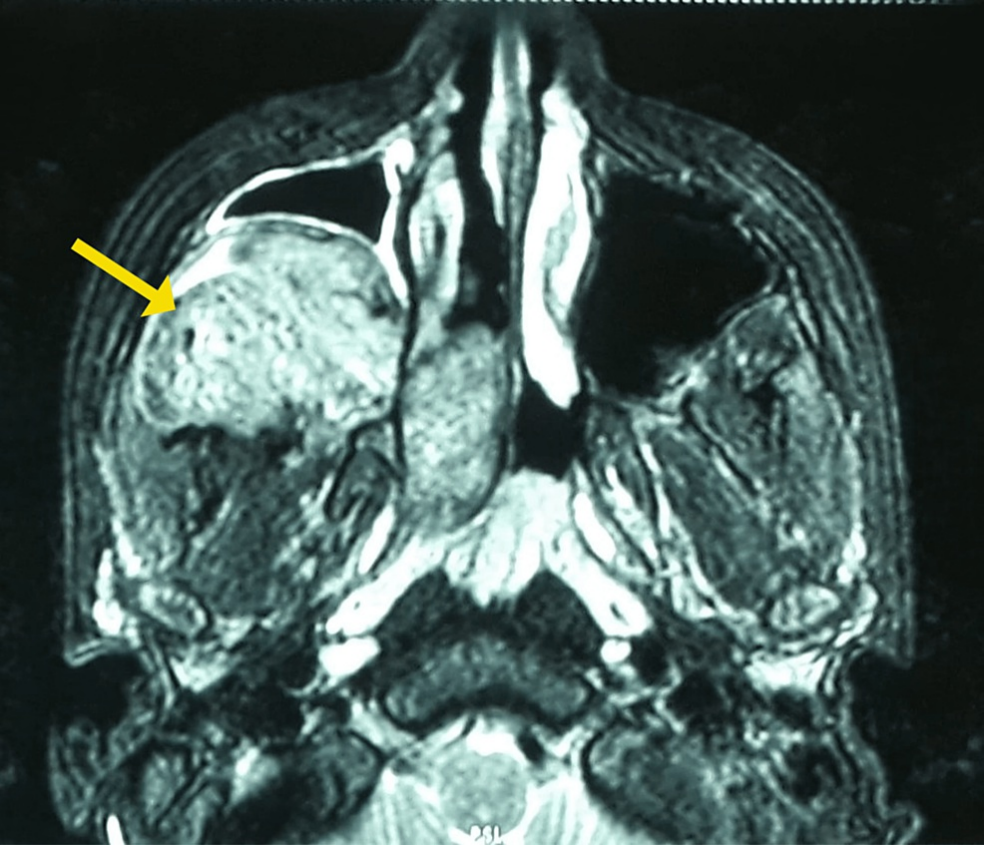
T1 weighted image showing a heterogeneously hypointense mass lesion occupying the right maxillary sinus in magnetic resonance imaging of paranasal sinuses
